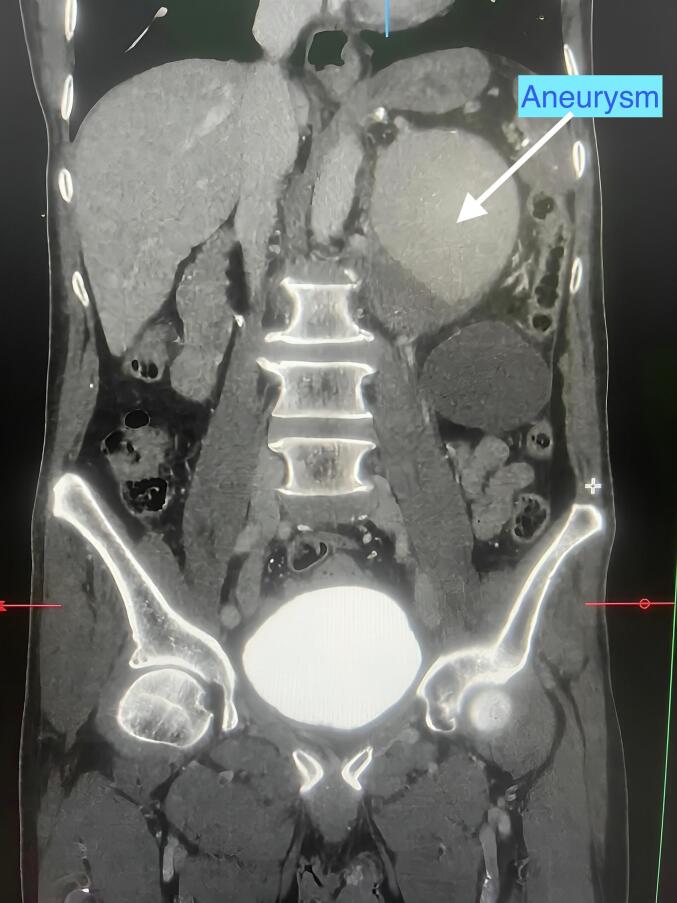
Contrast Enhanced CT of Abdomen showing Giant Splenic Artery Aneurysm
